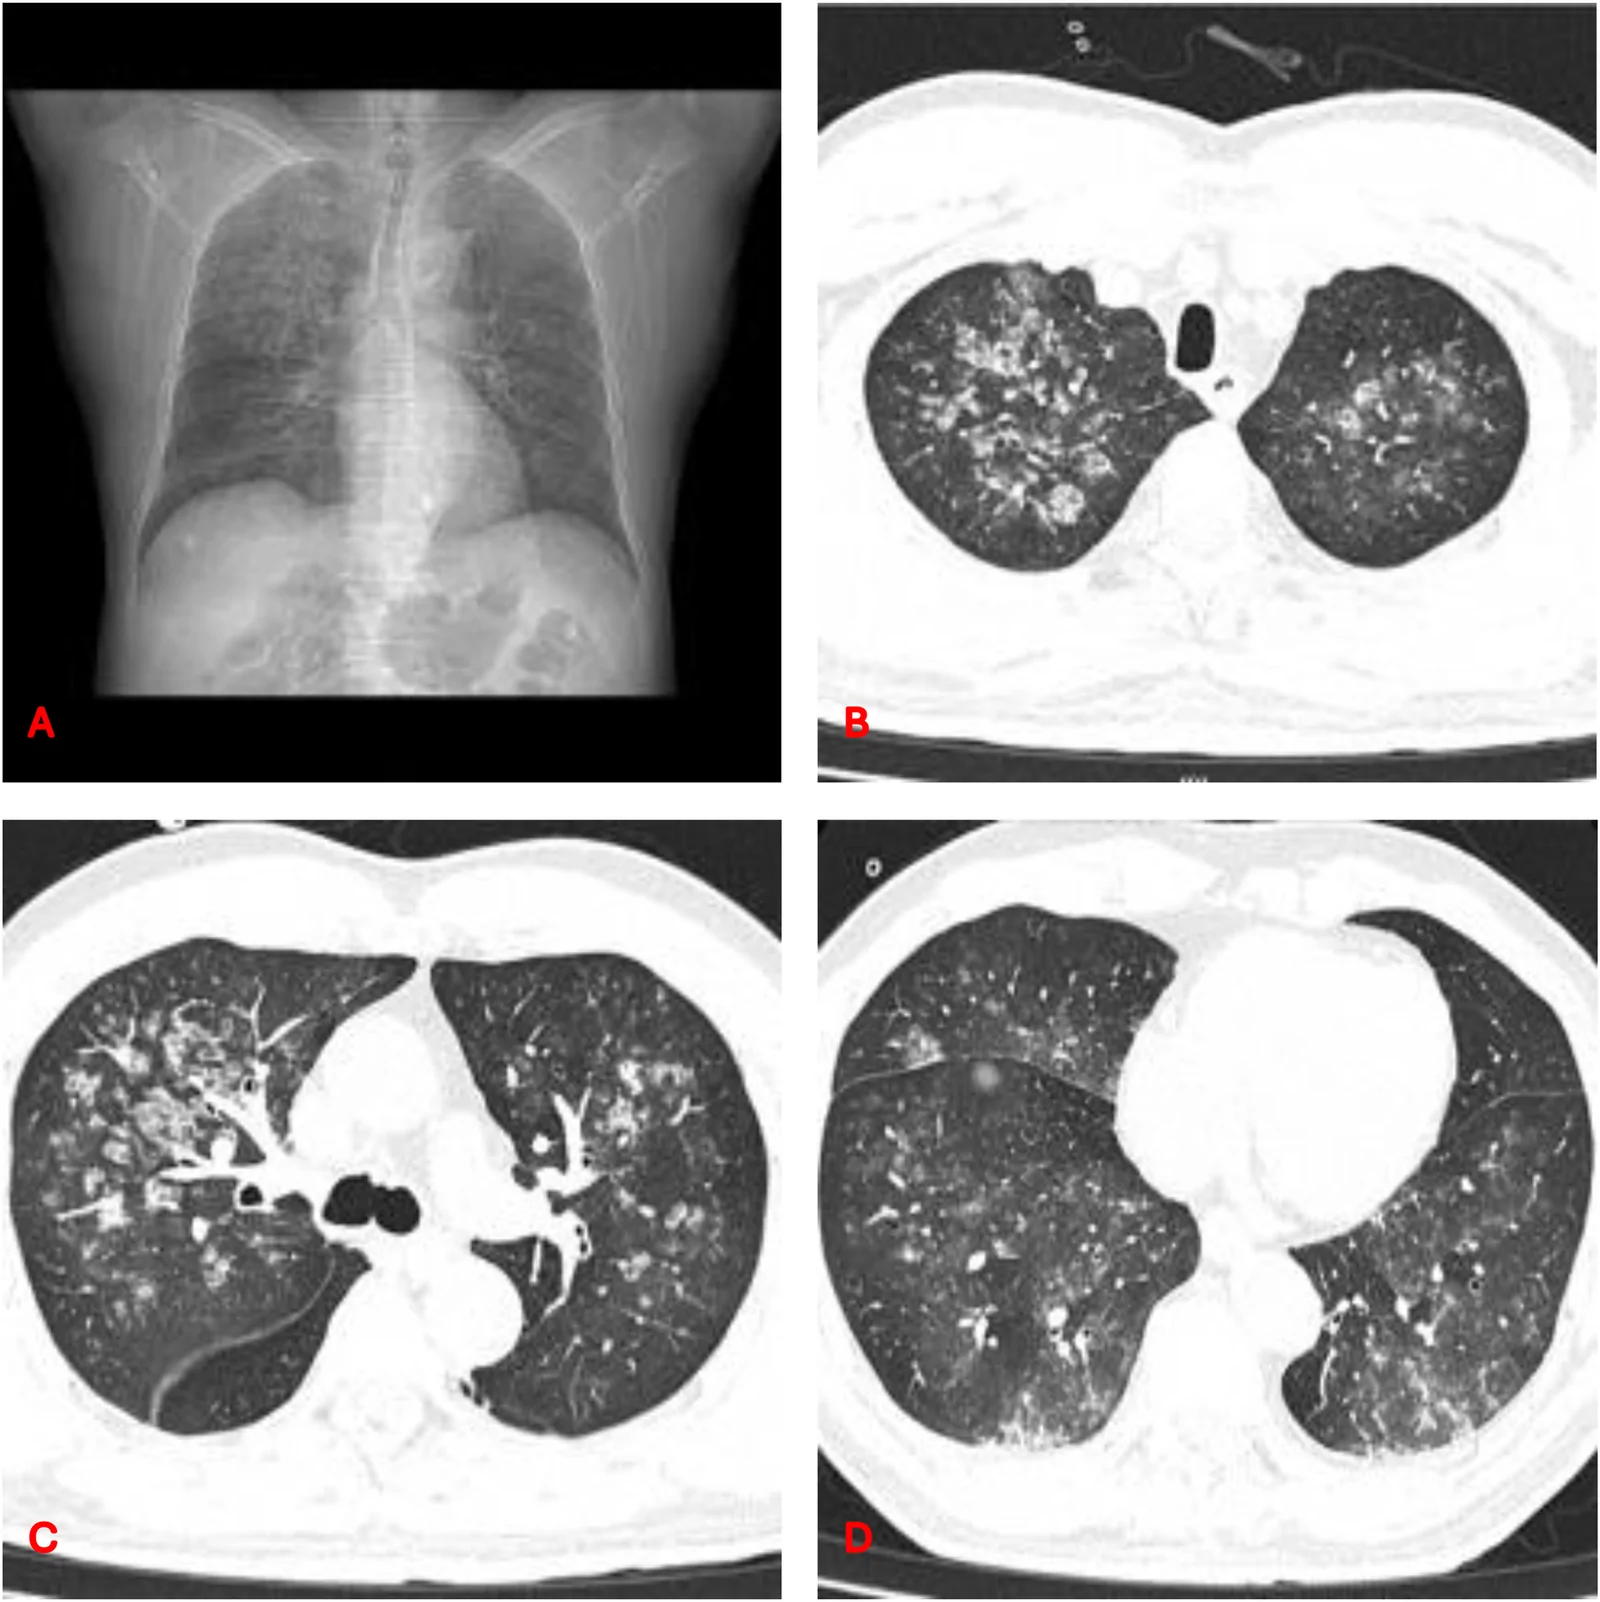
Initial chest x-ray and CT findings before treatment in chlorine inhalation–induced ARDS. (A) Chest x-ray on admission. (B–D) Corresponding chest CT images obtained on admission.
